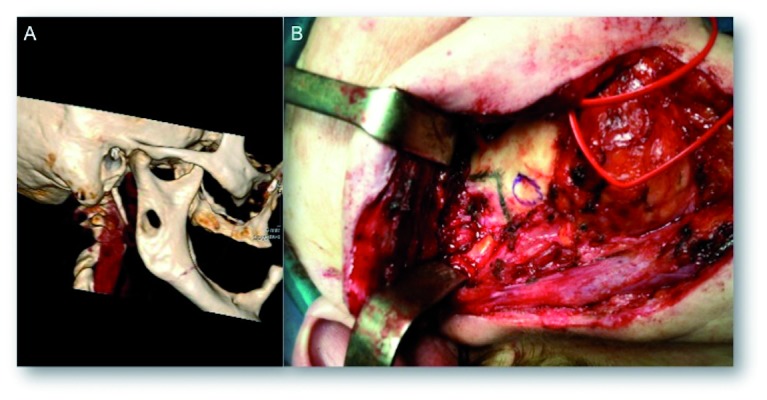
A. 3D CT image showing osteolytic lesion; B. Intraoperative view showing destruction of the buccal cortical bone of the mandible produced by the tumor.
